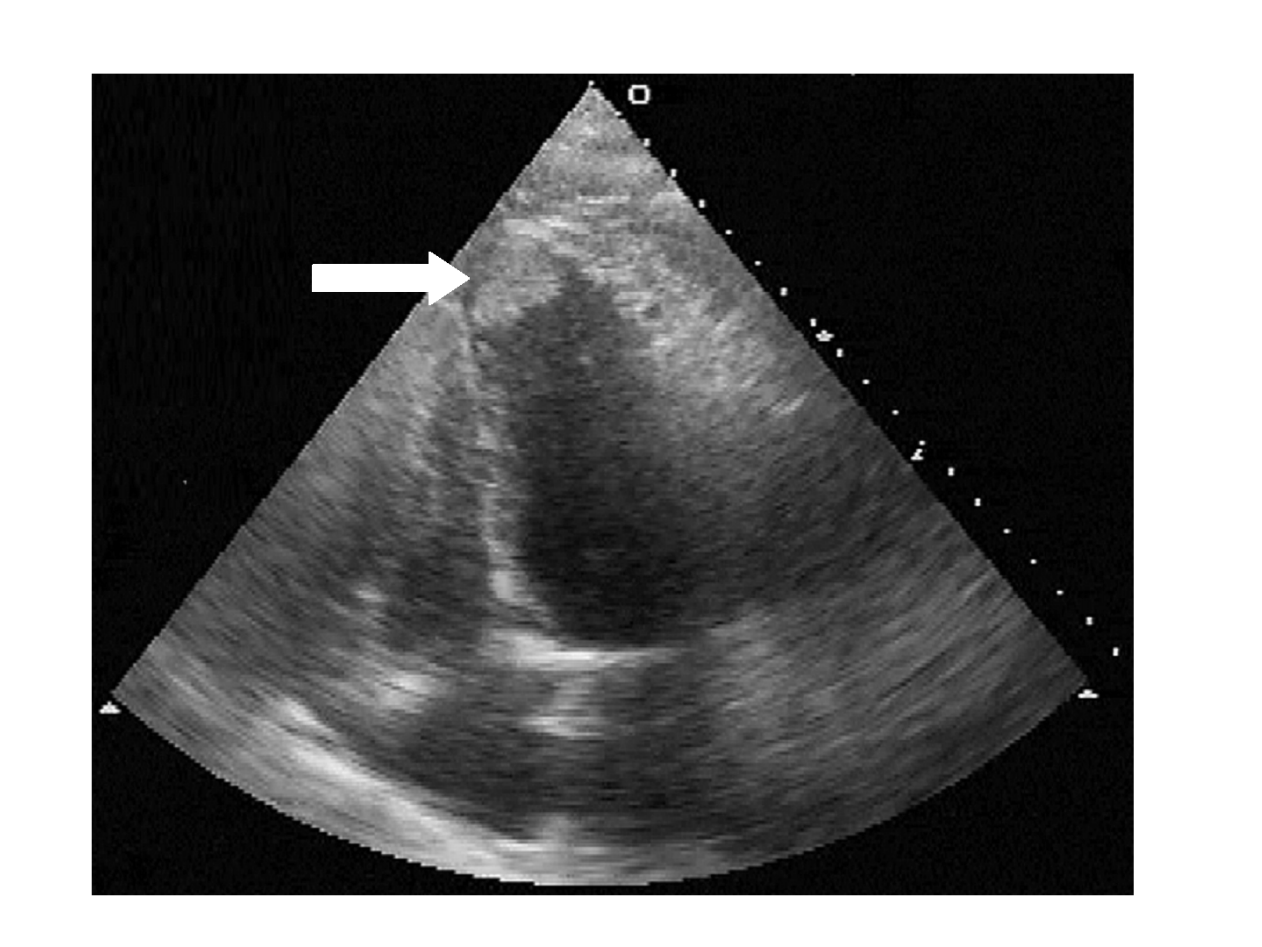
Cardiac duplex ultrasound image (4-chamber view), obtained 2 days after thrombolysis showing the thrombus attached to left ventricular apex (arrow).
